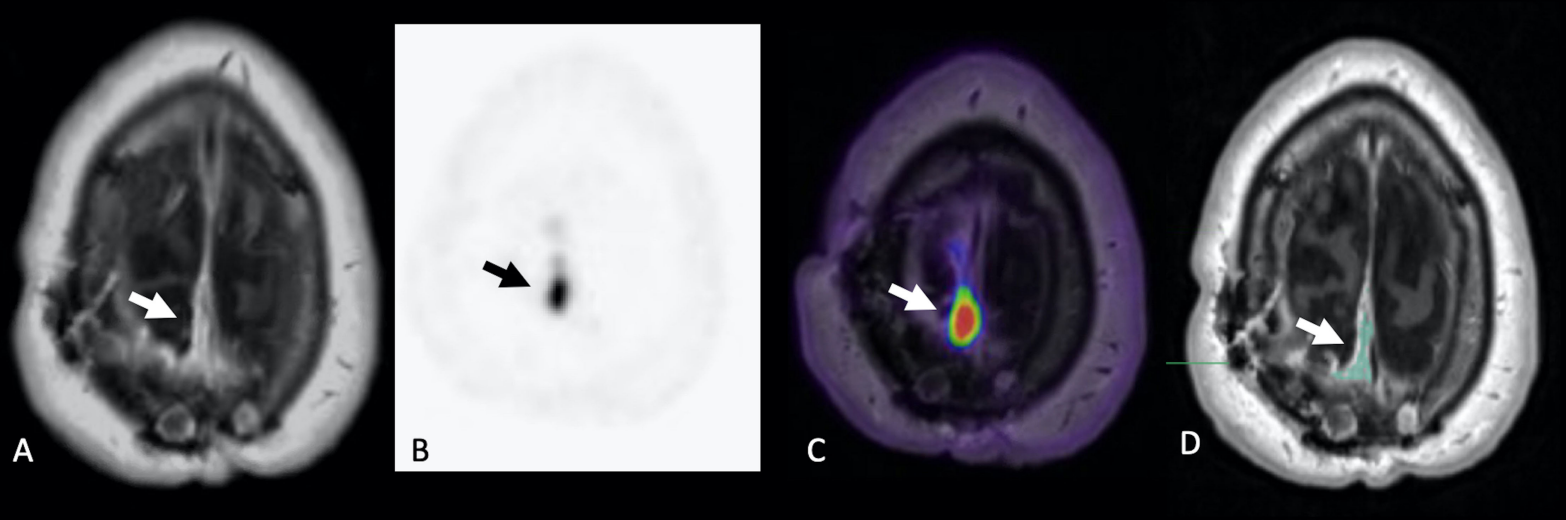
Example of VOI segmentation for DCE analysis in a 56-year-old man with WHO-2 right parietal meningioma post prior surgical resection and RT. Gadolinium-enhanced axial T1-weighted MRI (A), static 68-Gallium-DOTATATE PET image (B), and fused axial PET/MR (C) demonstrate extra-axial homogeneously enhancing soft tissue with corresponding 68-Gallium-DOTATATE avidity (arrows), compatible with residual/recurrent meningioma. Gadolinium-enhanced axial T1-weighted MRI with volume of interest (D) is shown, corresponding to region of suspected residual/recurrent meningioma.
